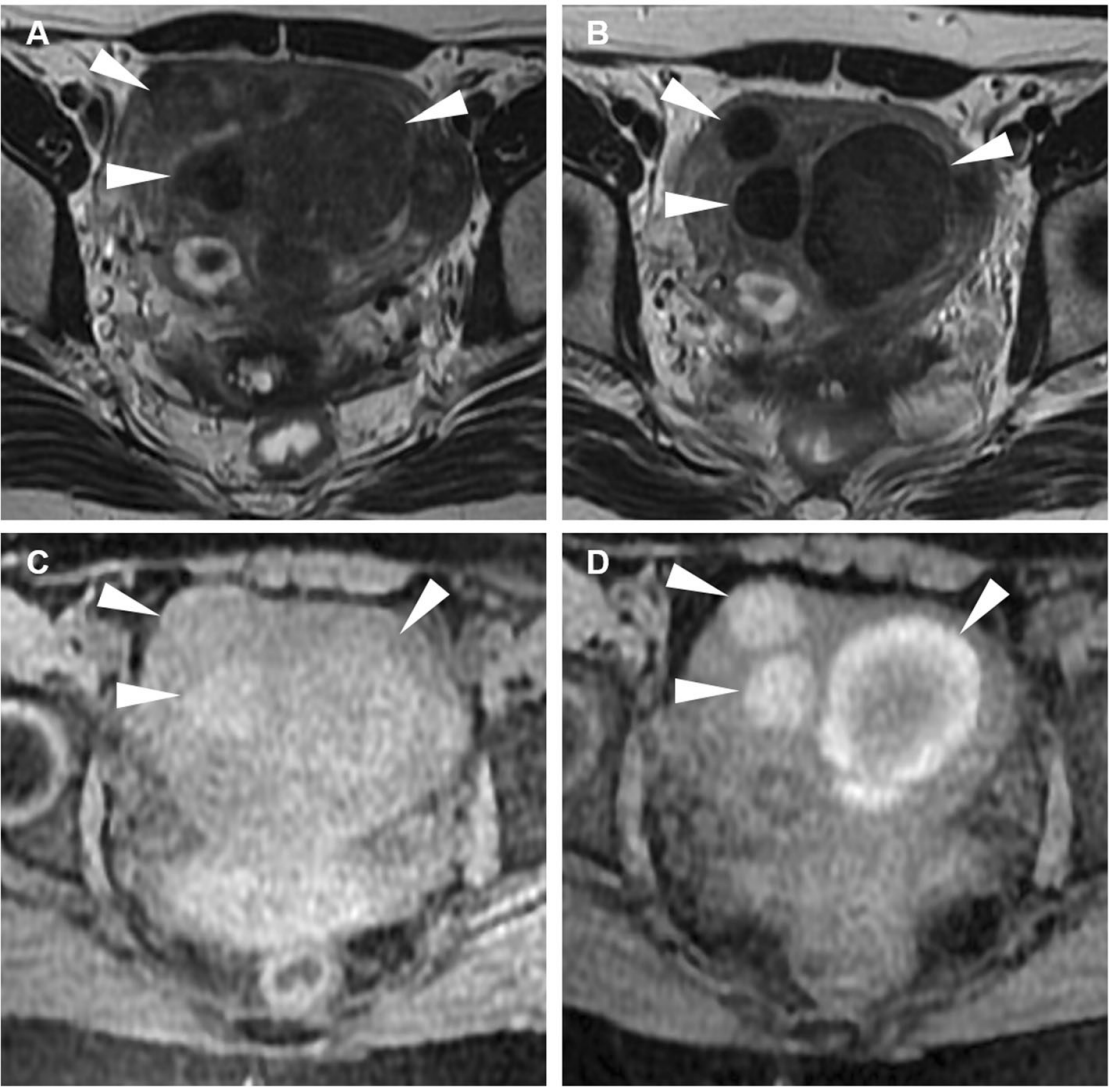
Multiple leiomyomas in a 48-year-old woman with pelvic pain and fever after uterine fibroid embolisation (UFE). Pre-treatment oblique-coronal T2-weighted (a) and fat-suppressed T1-weighted (c) images show multiple intramural leiomyomas, with respectively low and intermediate signal intensity (arrowheads). After bilateral UFE, on oblique-coronal T2-weighted (b) and fat-suppressed T1-weighted (d) images, the leiomyomas (arrowheads) show low T2 signal intensity and peripheral or homogeneous high signal intensity on fat-suppressed T1-weighted image reflecting internal haemorrhagic necrosis
